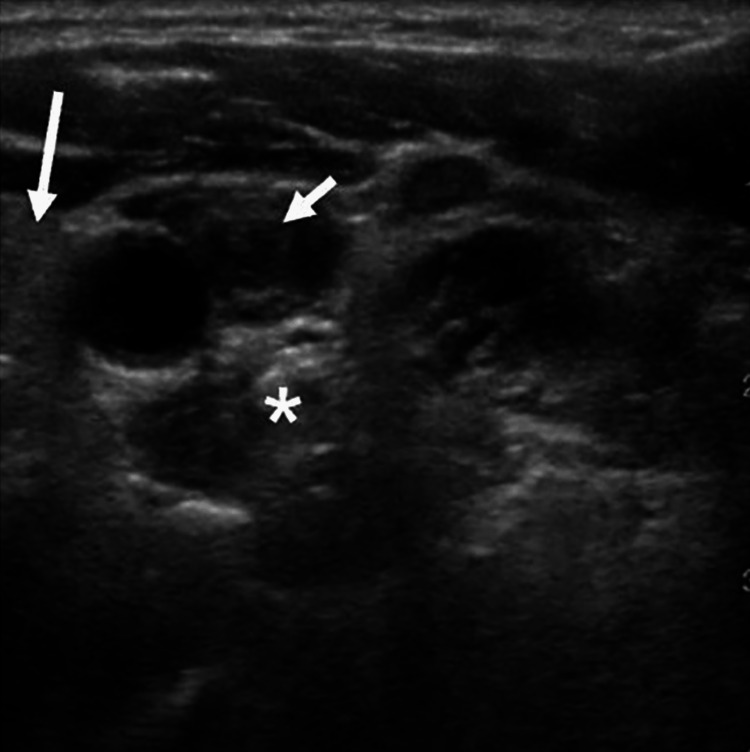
Preoperative ultrasound of the left neck demonstrating significant thickening of the vagus nerve (short arrow) and cervical sympathetic plexus (asterisk). Left thyroid (long arrow).
